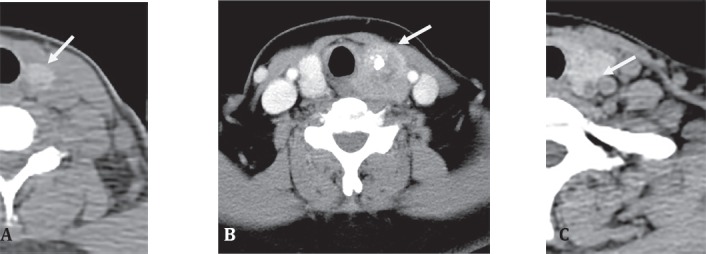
CT scans of thyroid nodular disease. A, B, Papillary carcinoma showing a hyperattenuating mass in the left lobe of the thyroid and a complex mass with calcification in the left lobe of the thyroid (*Image courtesy Prof. Dr. Jun Hatazawa, Osaka University Graduate School of Medicine*) c, benign adenoma seen as a complex mass deep within the left lobe of thyroid (*Image courtesy Prof. Dr. Henry Bom, Hwasun, Korea*)
